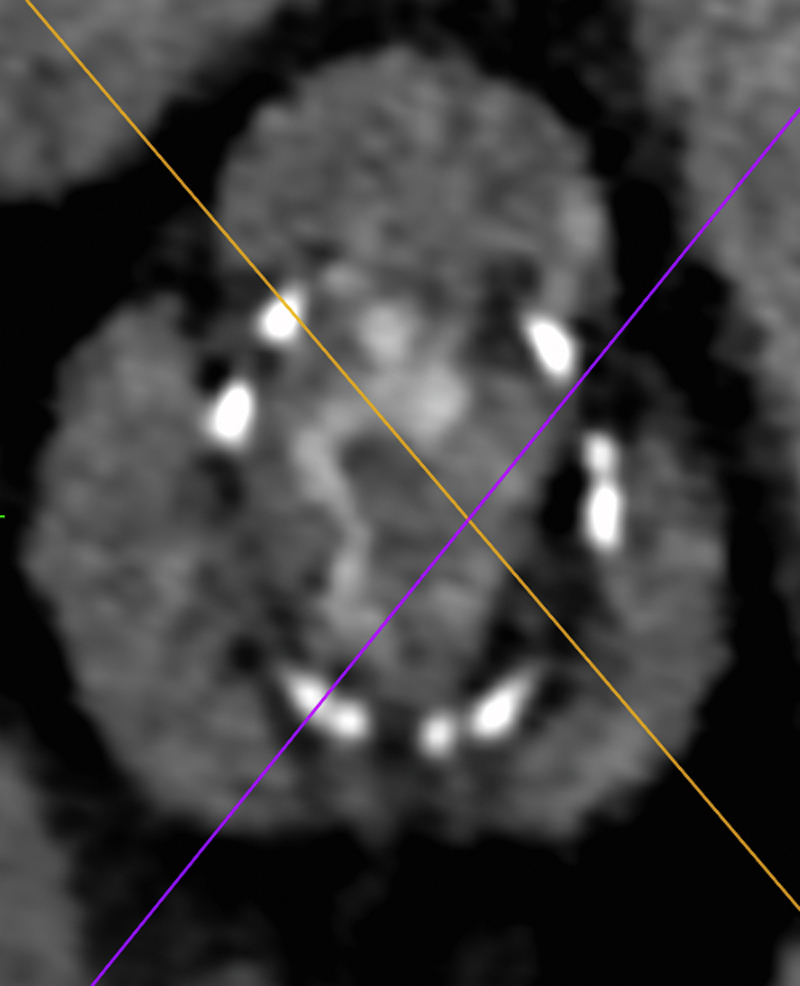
Computed Tomography Image at the Plane of Failed Surgical Bioprosthesis Transverse plane at the level of valve’s annulus showing that coronary ostia were not affected by surgical bioprosthesis encumbrance and testifying a very low risk of coronary occlusion.
